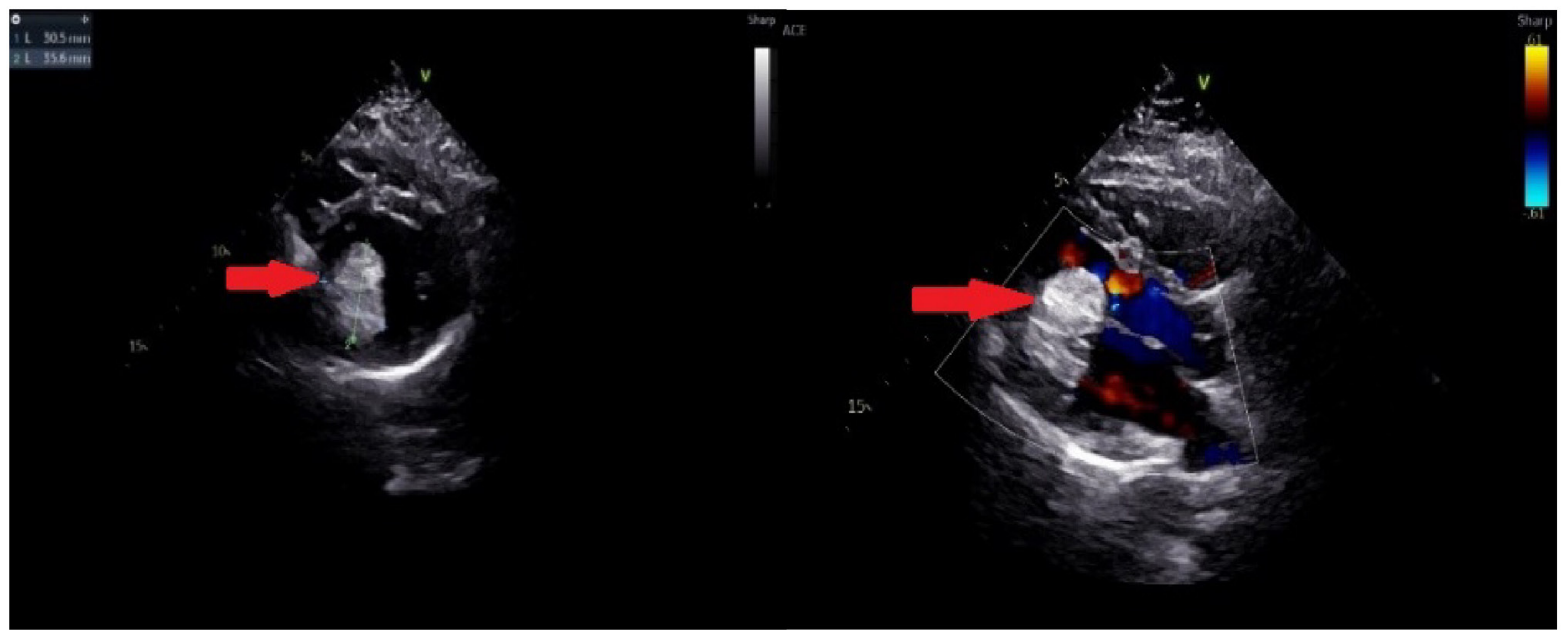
Echocardiography in the left ventricle. The arrow indicates an intracardiac tumor in the left ventricle.
